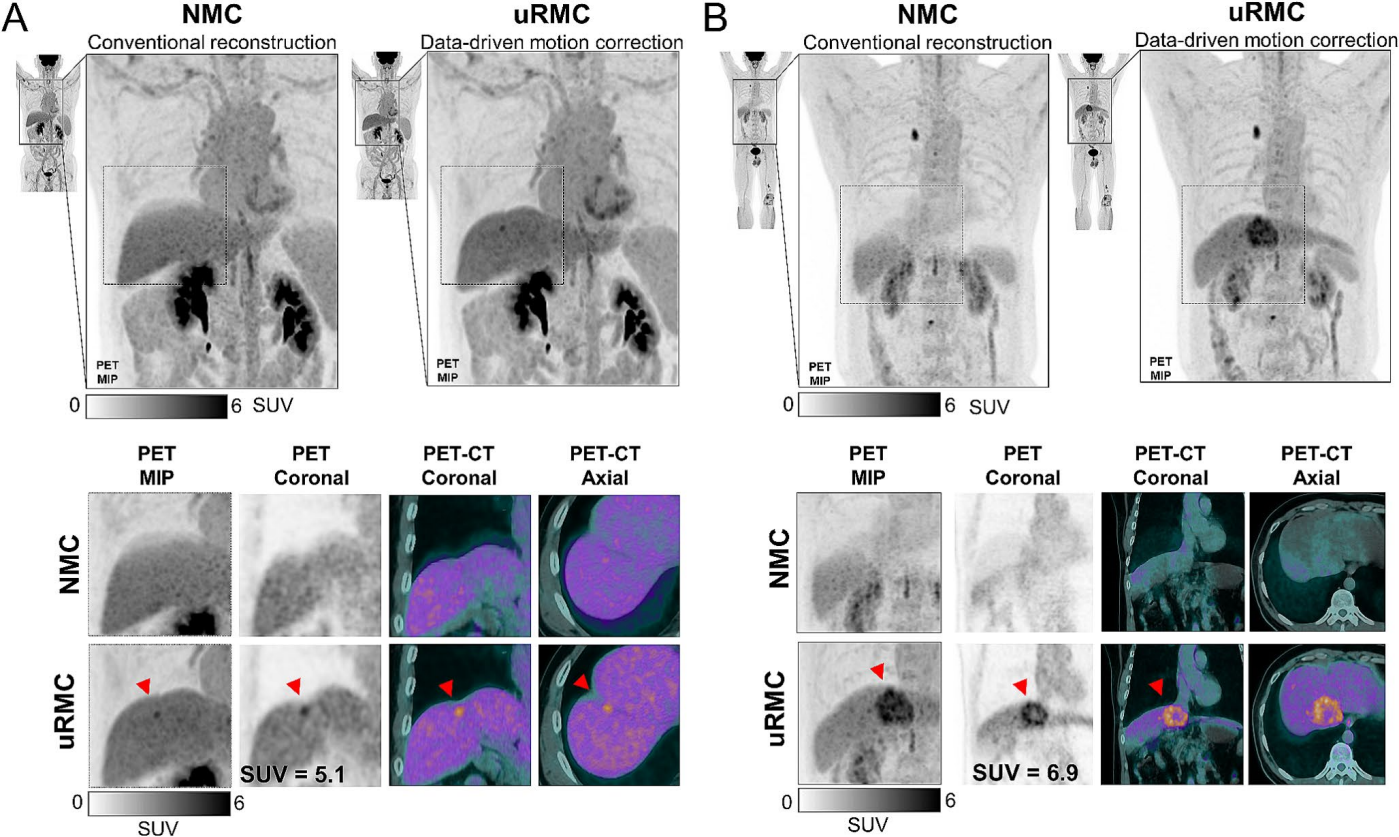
Examples showcasing potential additional diagnostic benefits provided by uRMC. (A) In restaging a patient with hepatocellular carcinoma after surgical resection of the primary site, an [^18^F]FDG-avid hepatic lesion is revealed at the resection margin with uRMC, whereas a conventional NMC image shows a negative result. (B) During restaging a patient with a prior history of left knee osteosarcoma, CT was acquired at deep inspiration, which mismatches with the PET that was acquired with free-breathing. Results in attenuation artifacts presented as a “banana artifacts” at the liver dome in the NMC images. With the use of uRMC, an annular [^18^F]FDG-avid lesion was unveiled, indicating a metastatic lesion with necrosis. NMC: no motion correction; uRMC: unified respiratory motion correction
